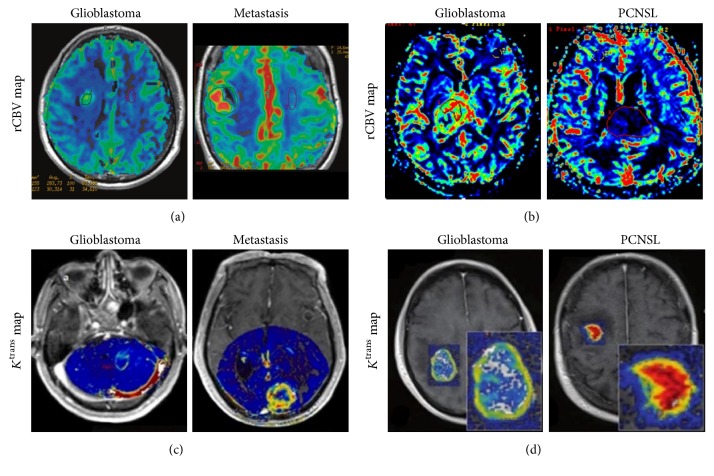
DSC-MRI (a) and DCE-MRI (b) for differentiation of GBM, PCNSL, and metastasis. rCBV maps demonstrate different characteristic features in the three distinct entities, with significantly higher rCBV value of GBM compared with metastasis and PCNSL. The *K*^trans^ value of GBM is significantly lower than metastasis and PCNSL. Reproduce with permission from Mangla et al. [118], Xing et al. [120], Zhao et al. [52], and Kickingereder et al. [121].
